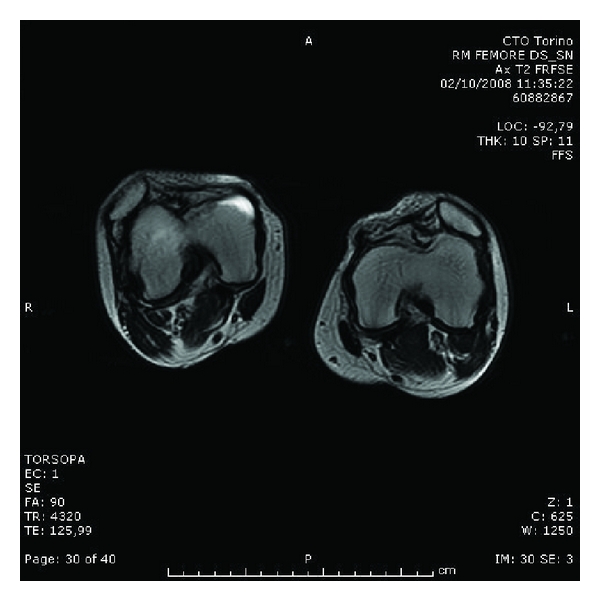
Significative MRI image of the knees (extended), showing the dislocation of the patellae, the severe hypoplasia of the medial side of the femoral quadriceps, and the pseudojoint of the lateral condyles.
